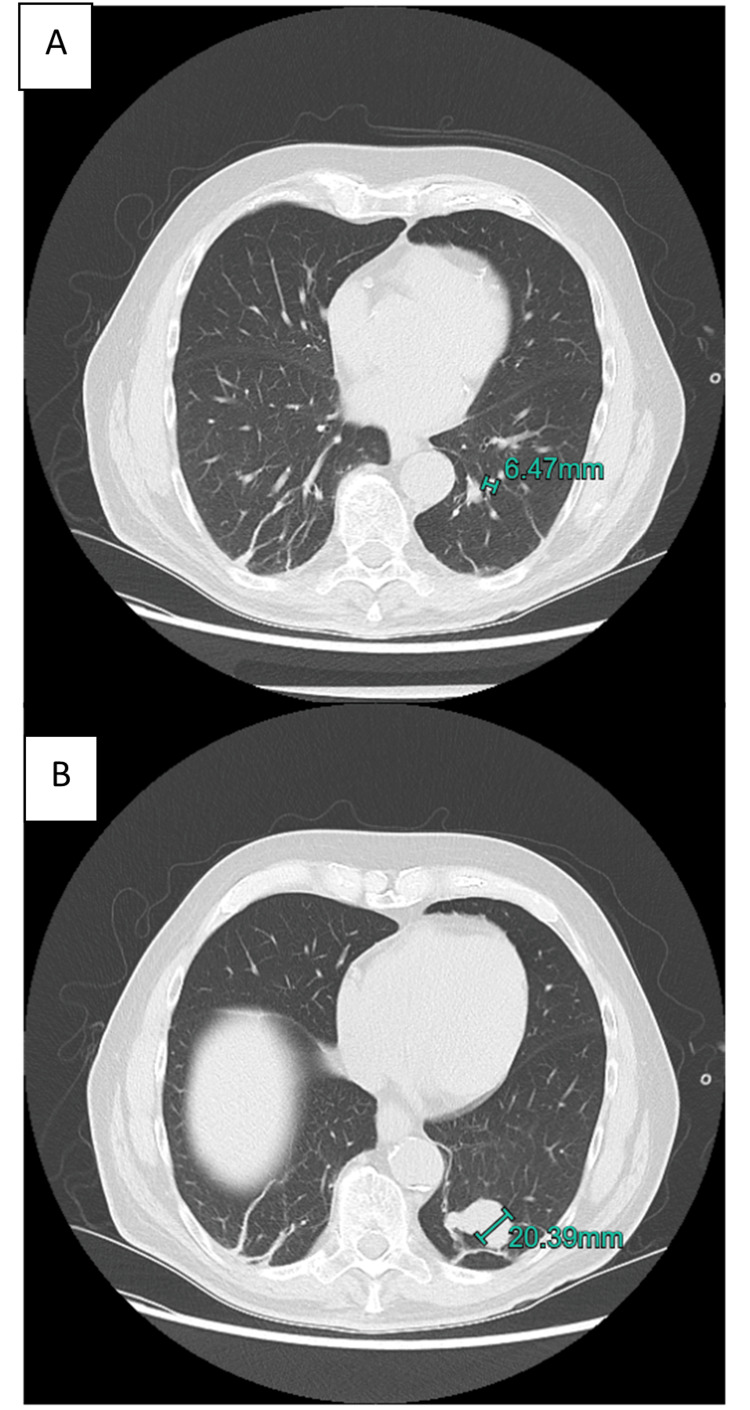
CT with intravenous contrast of the chest showing a pulmonary AVM A) Supplying artery measuring 6.47 mm; B) AVM with a maximum diameter of 20.39 mm. AVM, Arteriovenous malformation; CT, Computed tomography
